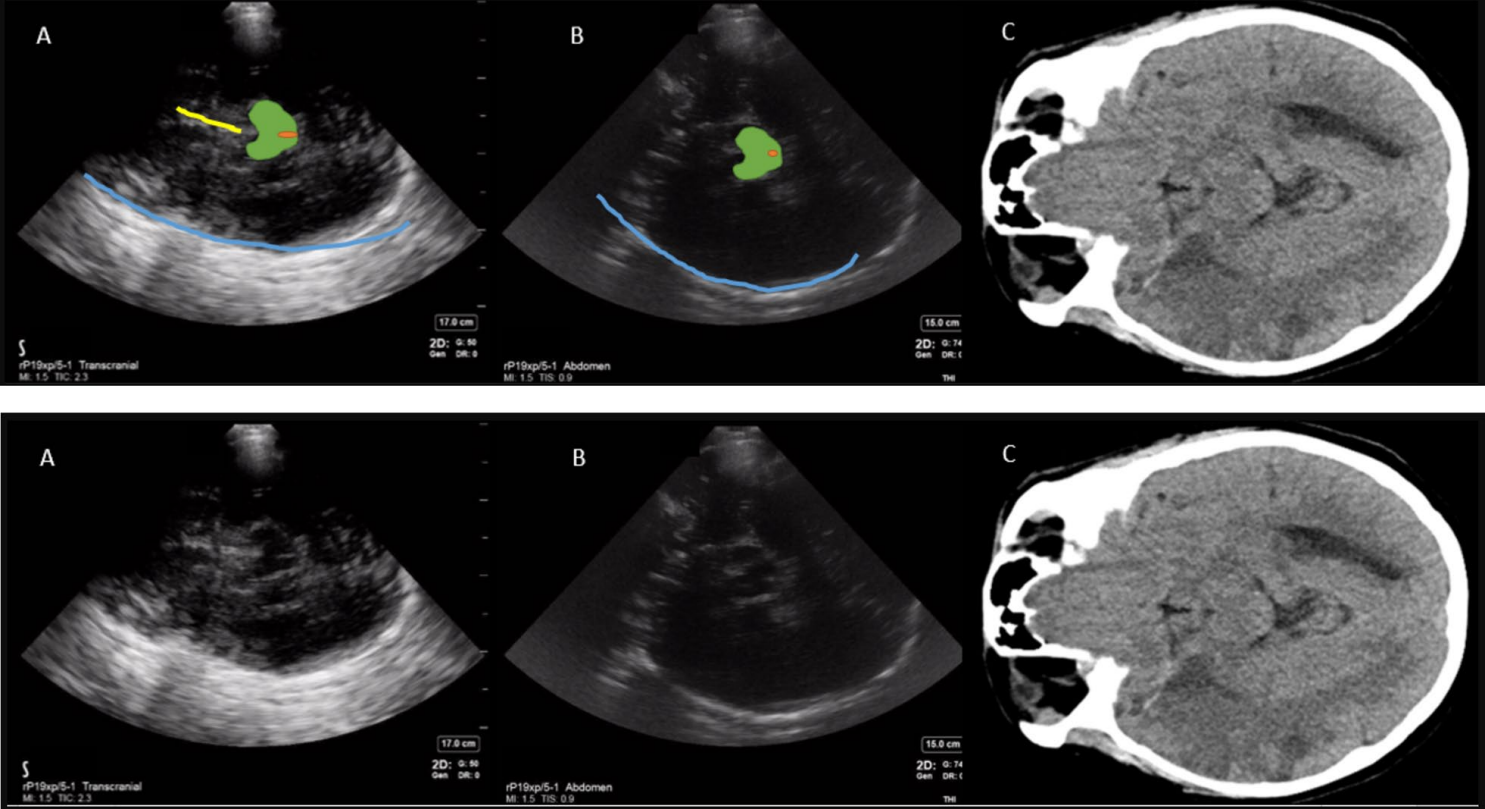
Acute ischemic stroke does not produce a characteristic appearance of ultrasound that allows ultrasound-based diagnosis. A—Transcranial preset, B—abdominal preset, C—computed tomography brain scan. Blue line—opposite skull, green midbrain, orange dot inside the midbrain—cerebral aqueduct, yellow line—falx cerebri
